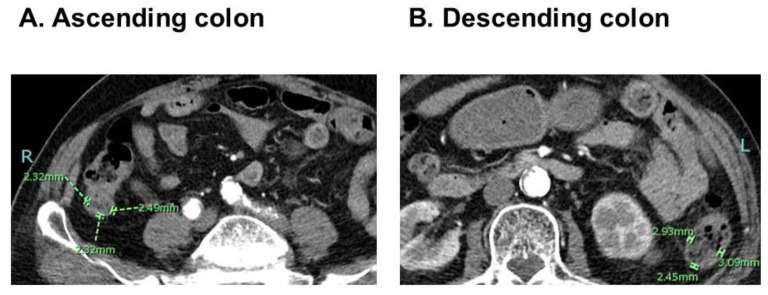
Measurements of CWT in the abdominal computed tomography. CWT was measured 3 times at the different positions of ascending colon (A) and descending colon (B), respectively. A total of 6 CWTs were obtained and averaged. In this representative case, the averaged CWT was calculated as 2.6 mm.
